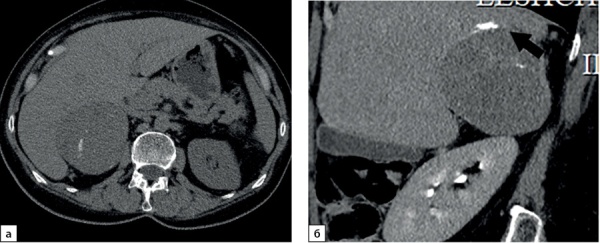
Рисунок 8. Компьютерные томограммы пациентки Л., 63 лет с опухолью правого надпочечника размерами до 72 мм, с полиморфными кальцинатами в структуре (стрелка): а — изображение в аксиальной плоскости, нативная фаза сканирования; б — фаза отсроченного сканирования, изображение реформатированное в сагиттальной плоскости.Figure 8. Computed tomography of patient L., 63 years old, with a tumor of the right adrenal gland up to 72 mm in size, with polymorphic calcifications in the structure (arrow): a — image in the axial plane, native scanning phase; b — delayed scan phase, image reformatted in the sagittal plane.
